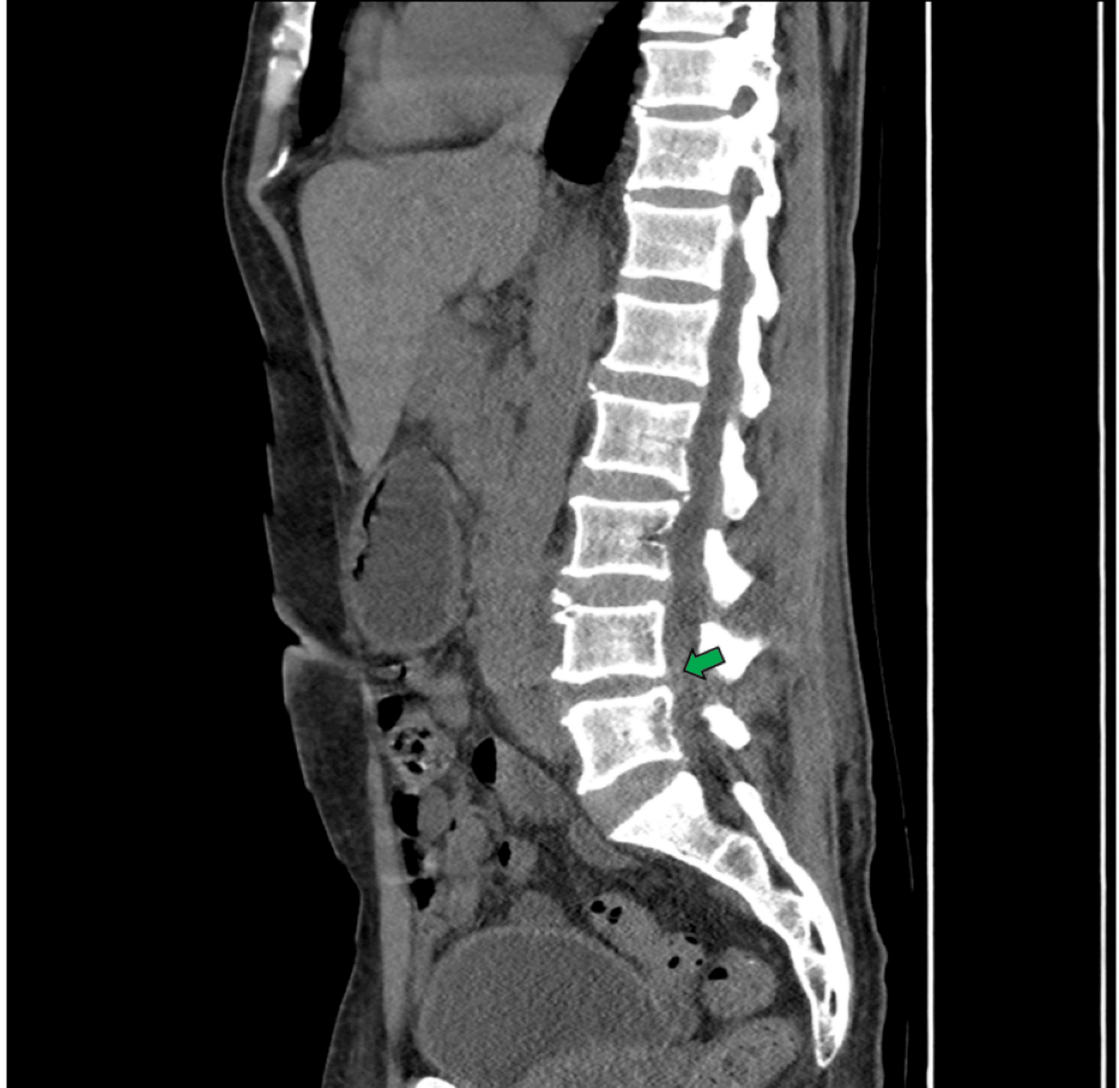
Abdominopelvic CT scan, sagittal view. The green arrow indicates degenerative changes in the lumbar spine, characterized by deforming spondylosis and L4-L5 intervertebral osteochondrosis. CT, computed tomography.
